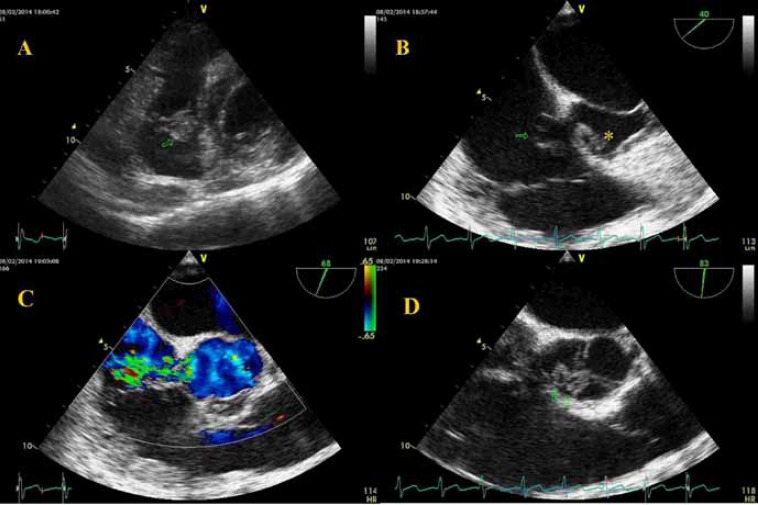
Echocardiographic views of the patient:
